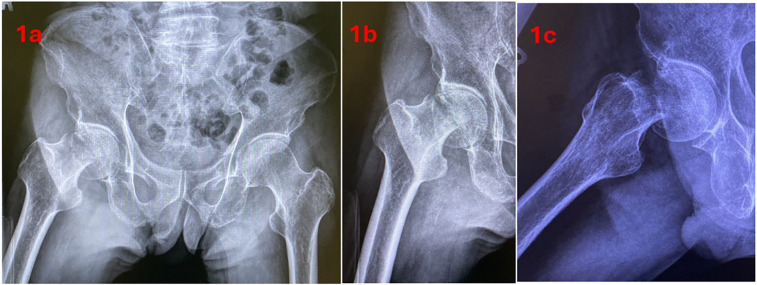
Pre-operative radiographs; (a) anteroposterior pelvis view, (b) anterior hip view, (c) lateral hip view.
